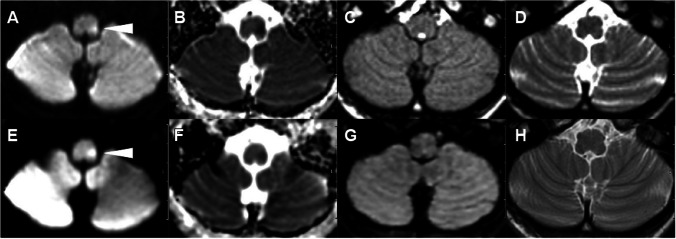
Images in a 55-year-old woman who presented with left-sided weakness and posterior circulation symptoms (NIHSS 10). CT without infarction but thrombosis of the left vertebral artery was shown on CT angiography. Axial DWI, ADC, T2-FLAIR, and T2-weighted images, A–D NeuroMix upper row and E–H cMRI lower row. A, E MRI performed 2.9 h after symptom onset shows a subtle DWI lesion to the left in the medulla oblongata (arrowhead). The DWI lesion has lower conspicuity on NeuroMix compared to cMRI. All readers rated the lesion as negative on T2-FLAIR corresponding to DWI/T2-FLAIR mismatch. The clinical radiological interpretation was acute infarction with DWI/T2-FLAIR mismatch, and treatment with alteplase was given after MRI with a reduction of symptoms (NIHSS from 10 to 2)
